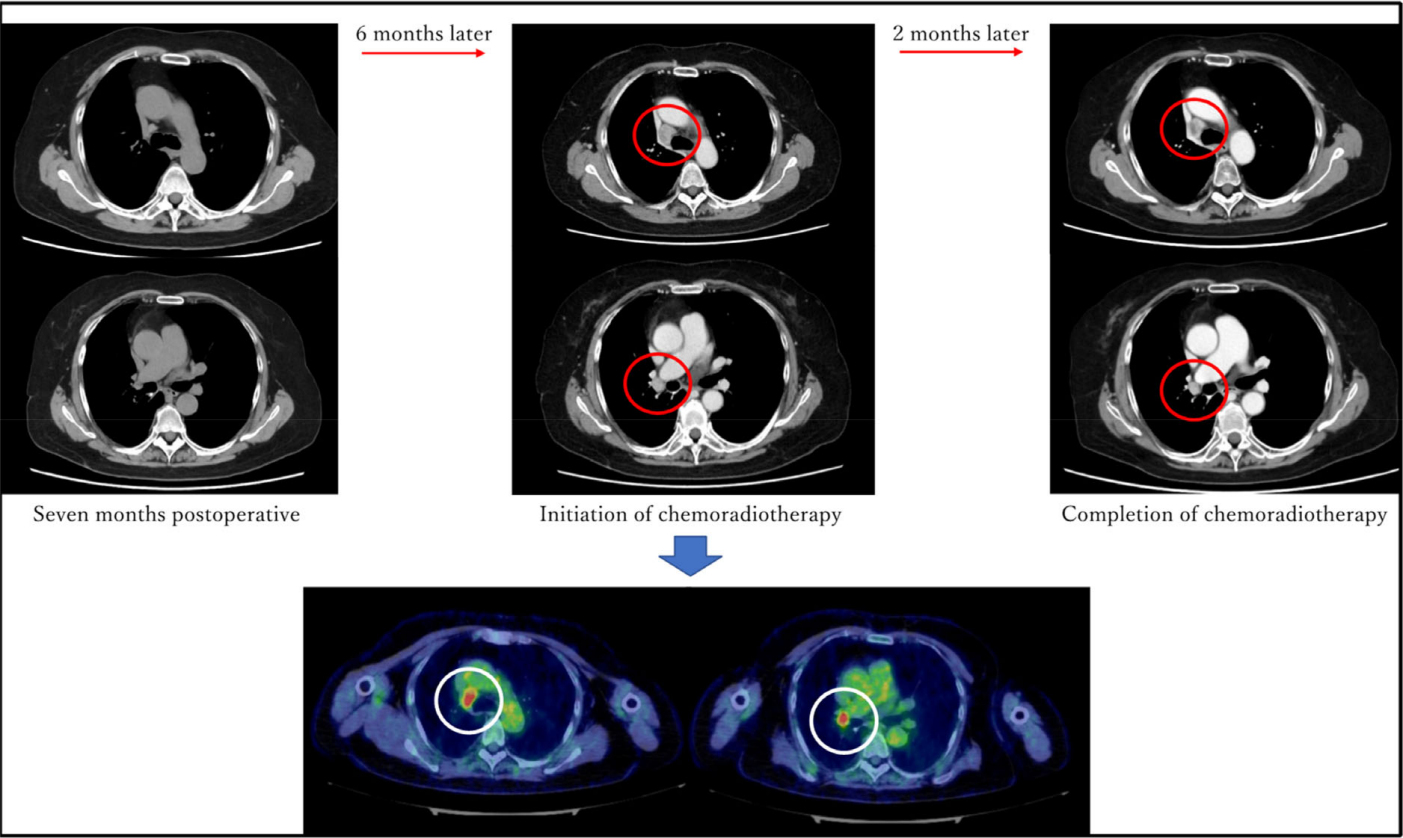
At 7 months postoperatively, no evidence of recurrent disease was seen on chest plain computed tomography (CT) (upper‐left). Chest contrast‐enhanced CT conducted ~6 months later showed the enlargement of two mediastinal lymph nodes (upper center). At approximately the same time, fluorodeoxyglucose positron emission tomography‐computed tomography (FDG/PET‐CT) showed the accumulation of FDG in the same region with an SUVmax of 4.9–5.58 (lower center). Chemoradiotherapy was administered to the same region, and at the time of completion, the disease was stable according to the Response Evaluation Criteria in Solid Tumors criteria (upper‐right).
